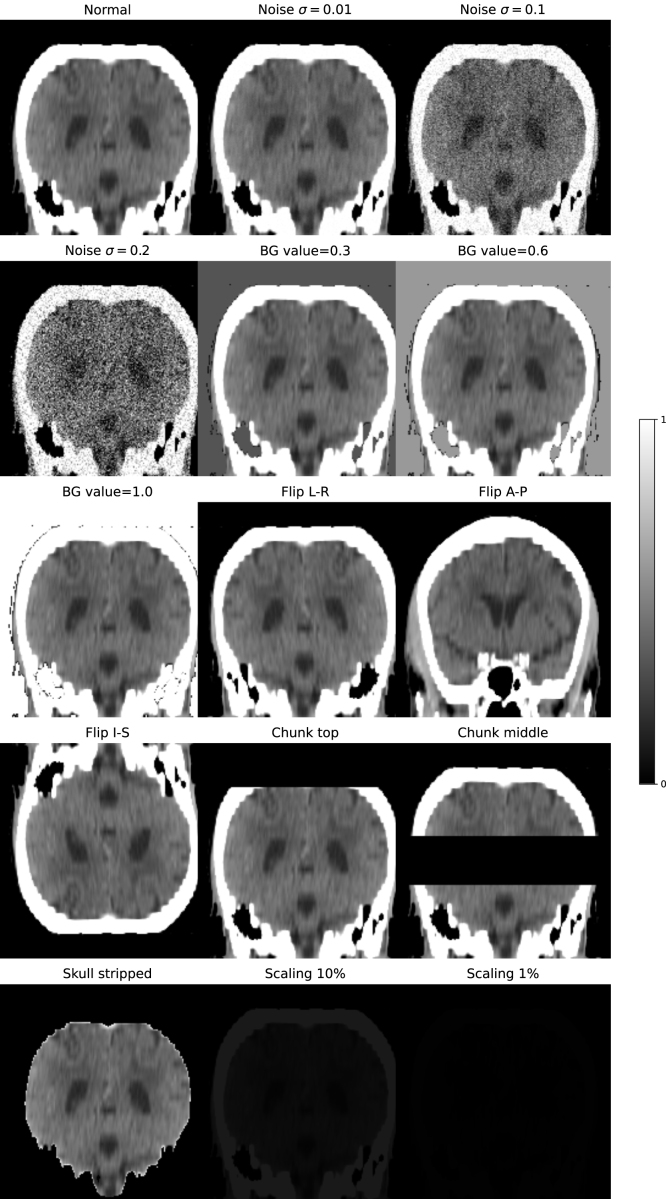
Example of all the corruptions applied to one subject from the KCH head CT dataset. All images are shown with the same intensity range. Corruptions are: Noise: Adding Gaussian noise $∼N\left(0,\sigma^{2}\right)$, BG value: replacing background value of 0 with a new constant value, Flip: invert image through described plane; Chunk: set a number of parallel slices = 0, Skull strip: remove skull, Scaling: reduce global image intensity by multiplying by a fixed factor.
